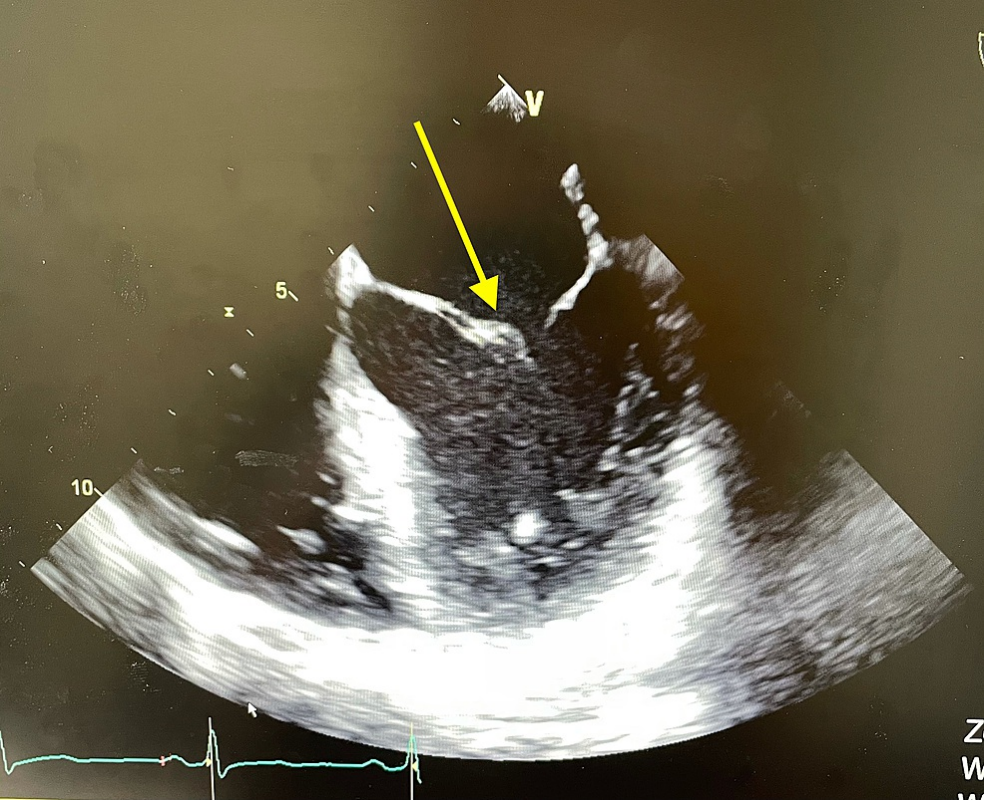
TEE showing a vegetation on the anterior mitral valve leaflet. TEE: transesophageal echocardiogram
